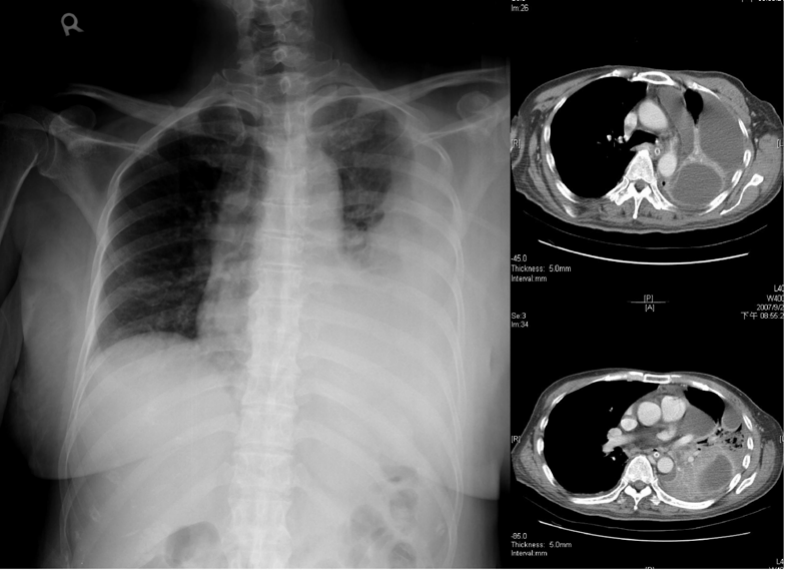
The chest radiograph and computed tomography scan showed pleural empyema without lung abscess.
